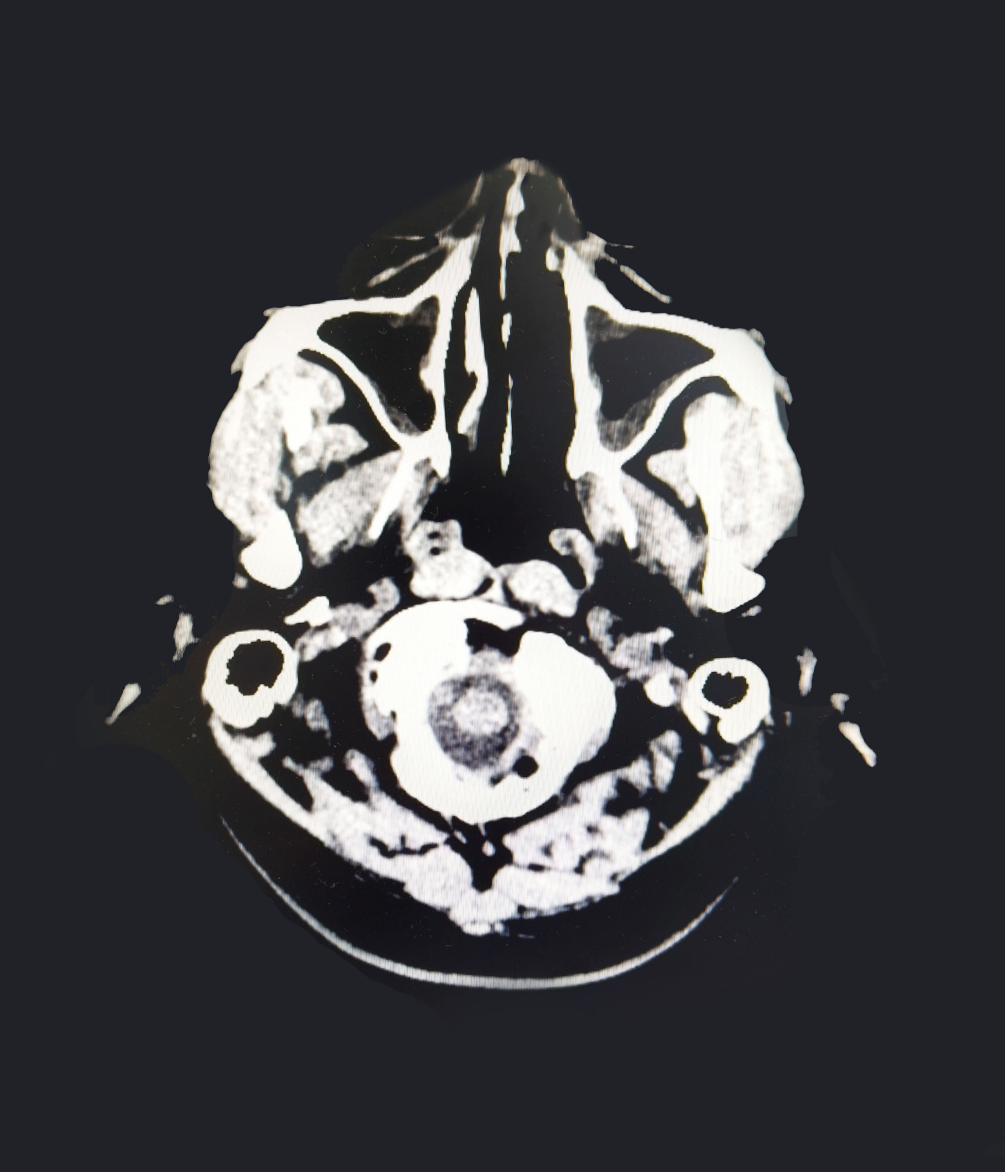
Paranasal sinus CT showing high density in the left nasal cavity.
